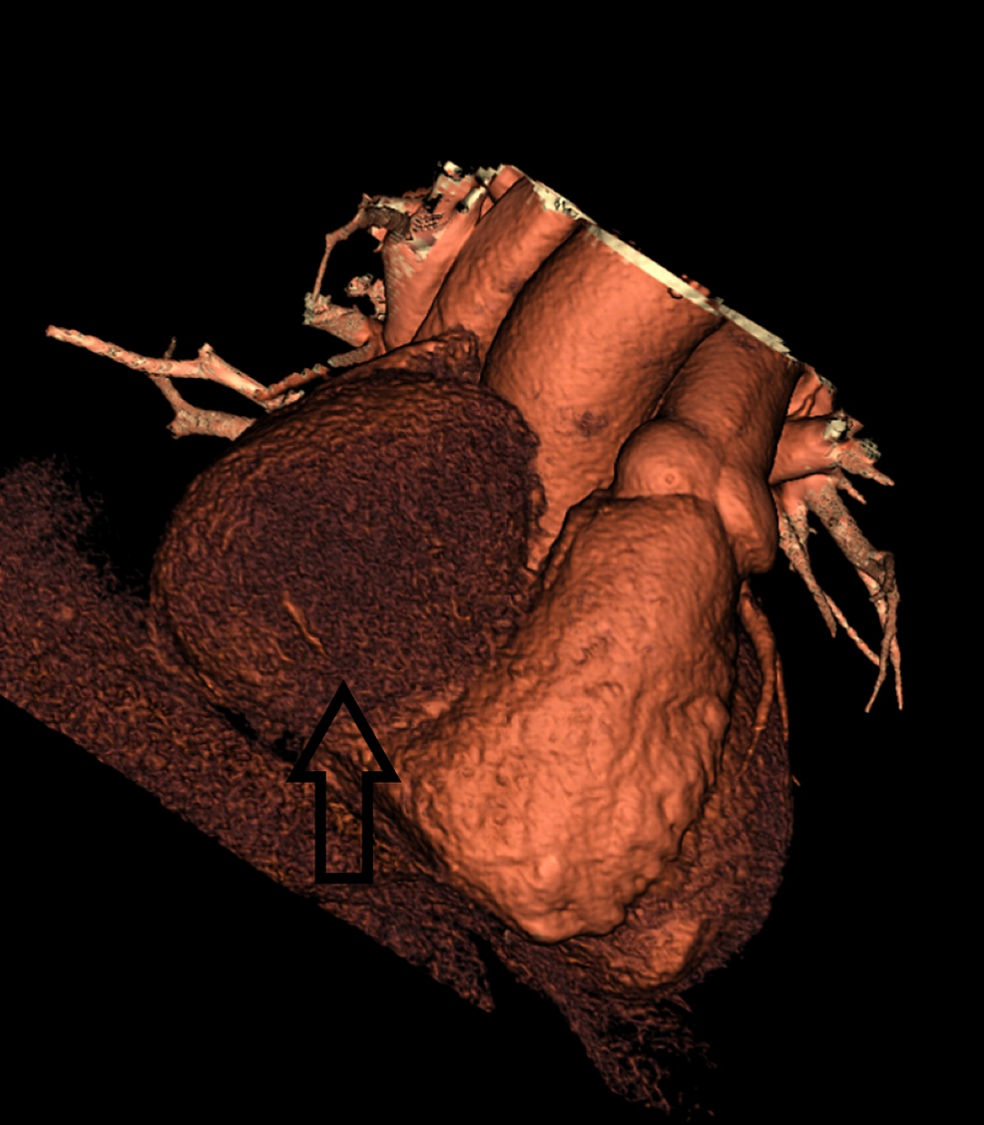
3D reconstructed CT heart with coronaries demonstrating the right coronary artery aneurysm.
